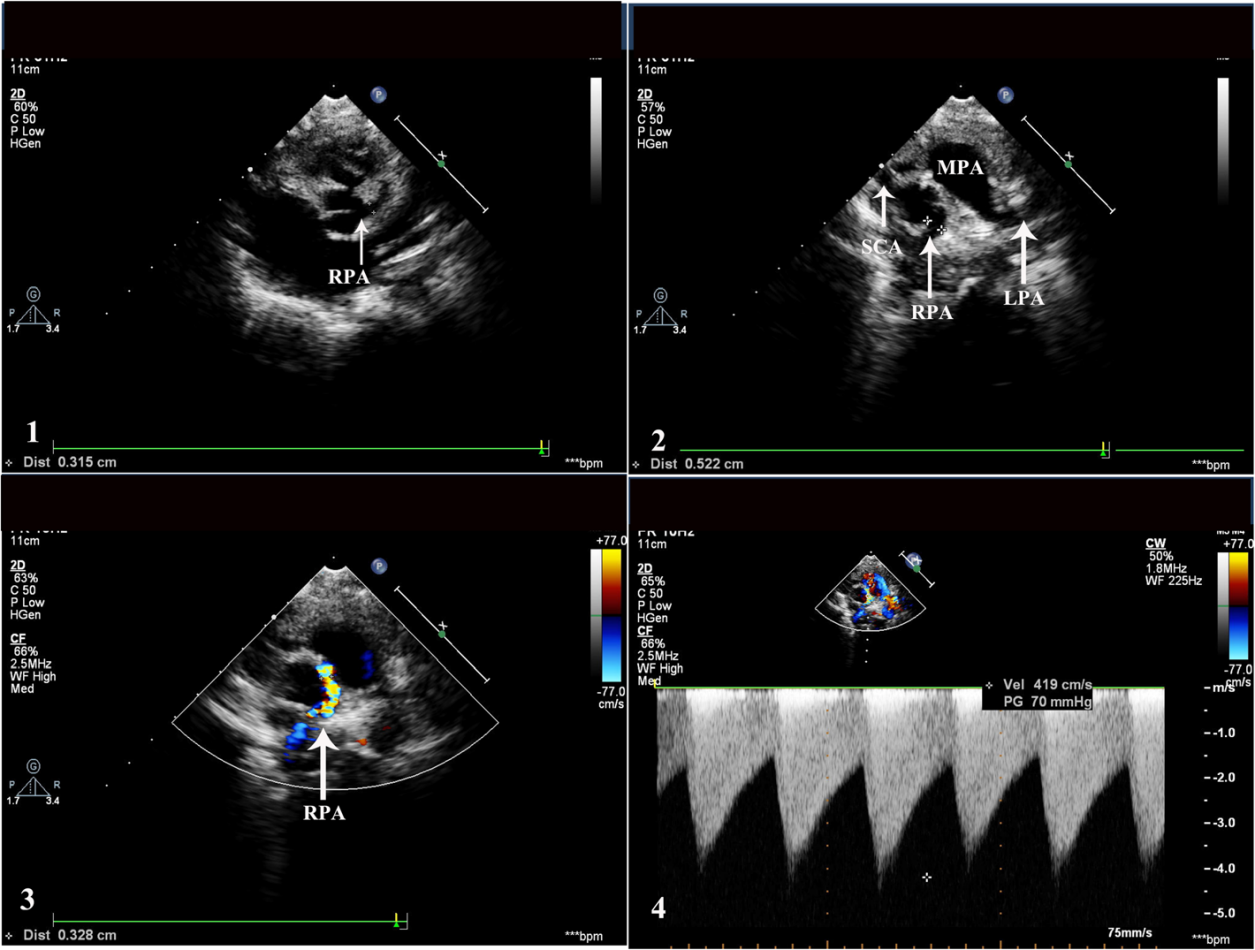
1 and 2: The reconstructive RPA originated from MPA, SCA arising from right aortic sinus in short axis view of echocardiography. 3: The color flow Doppler showed turbulent flow in RPA. 4: Continuous wave Doppler showed RPA blood flow velocity (4.19 meter/sec). SCA: single coronary artery; MPA: main pulmonary artery; RPA: right pulmonary artery; LPA: left pulmonary artery; LCx: left circumflex coronary artery; LAD: left anterior descending coronary artery; RV: right ventricle; PDA: patent duct arteriosus
